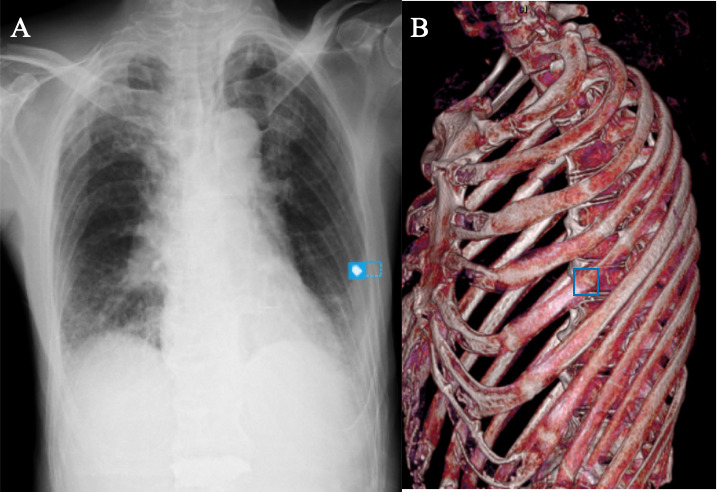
Representative true-positive rib fracture detected by artificial intelligence (AI) and confirmed by computed tomography (CT) during prospective emergency department deployment. (A) Chest radiograph showing an AI-flagged fracture of the left fifth rib that was not described in the initial radiology report. (B) Corresponding CT image confirming the fracture at the same anatomical location.
